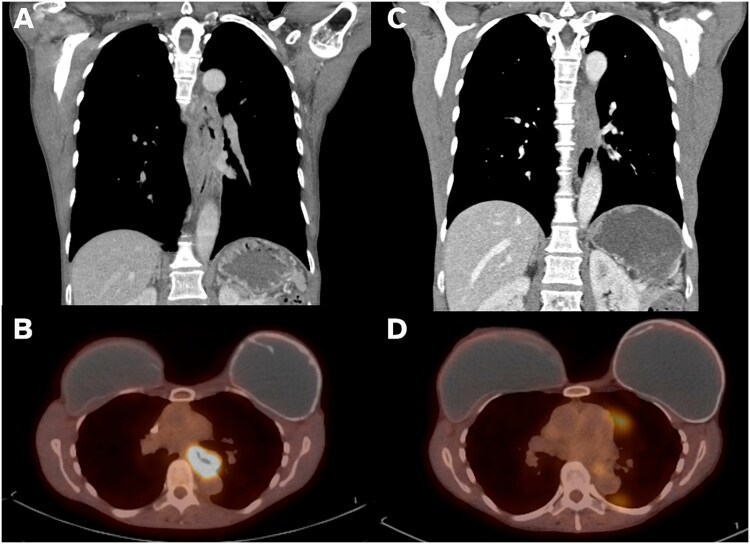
Imaging findings on presentation vs post-neoadjuvant therapy. (A) Coronal CT imaging showing 8.6-cm long esophageal mass. (B) PET imaging with mid-esophageal mass with SUV 15.4. (C) Post-CROSS protocol esophageal mass with significantly improved wall thickening on coronal CT. (D) Post-CROSS PET with decreased FDG avidity at the level of the mid esophagus.
